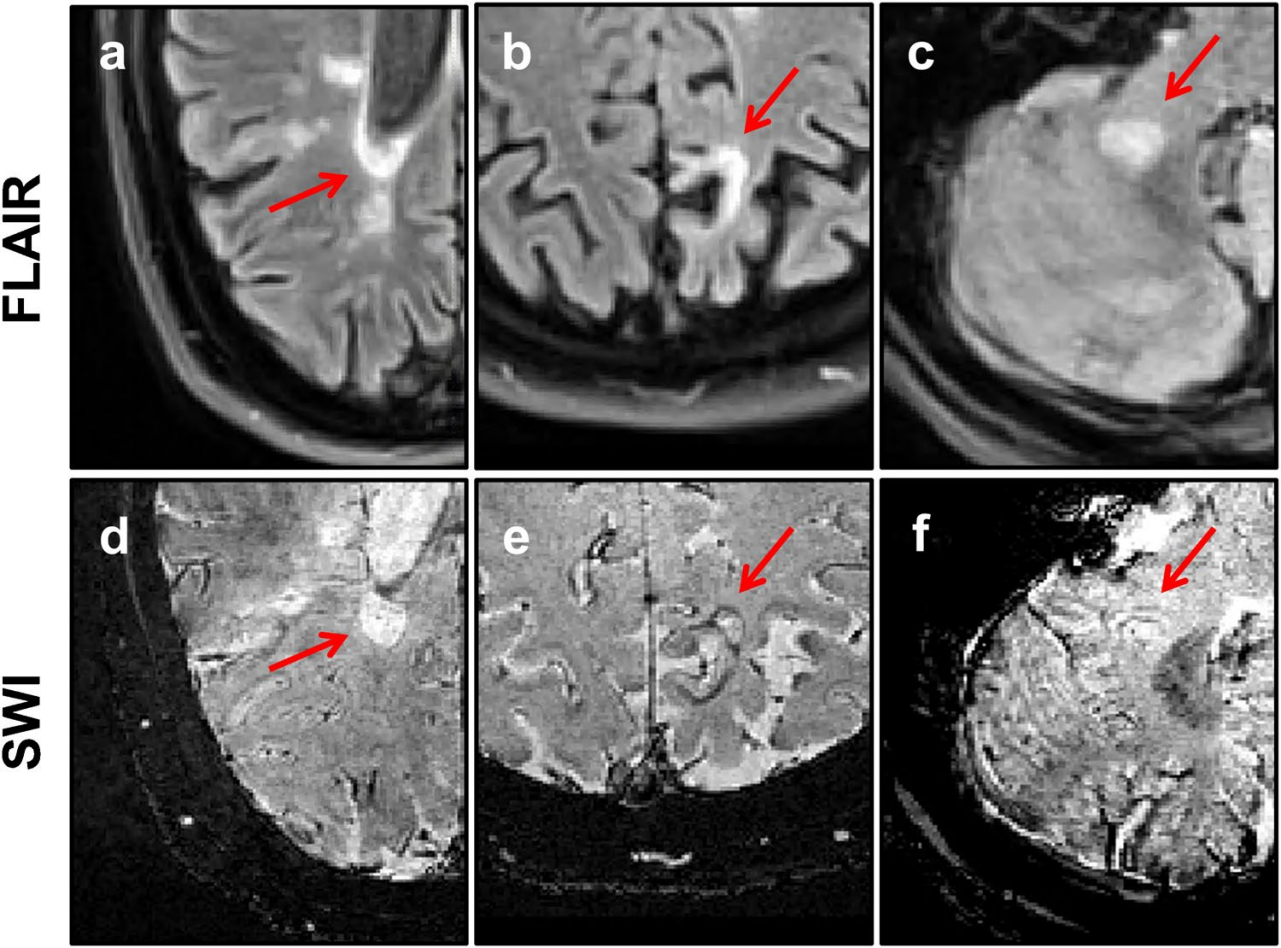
Rim lesions observed at 3 T. SWI and corresponding FLAIR images showing representative examples of rim lesions (indicated by red arrow) in different brain locations including periventricular (a, d), juxtacortical (b, e) and infratentorial (c, f) regions
